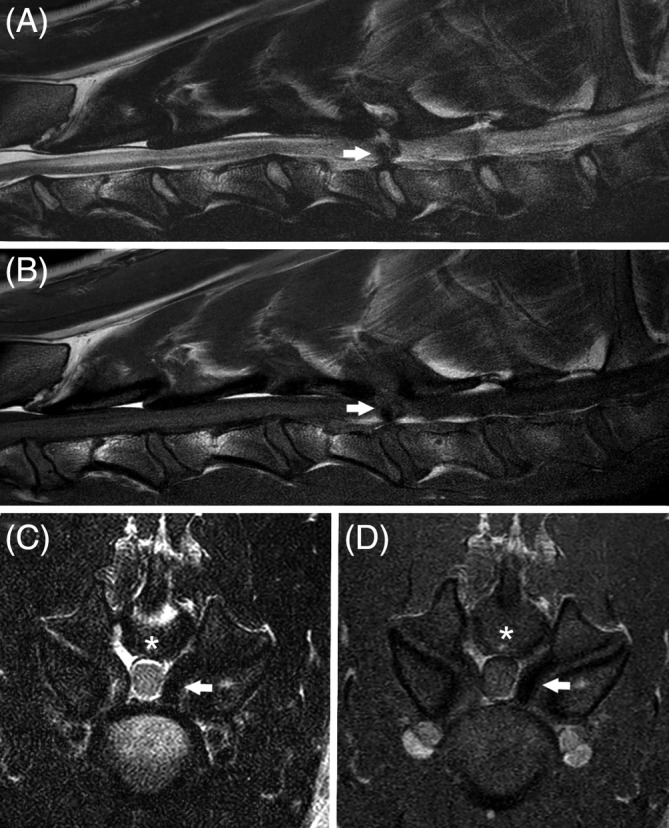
Parasagittal T2‐weighted (A) and T1‐weighted (B), transverse T2‐weighted (C) and T1‐weighted (D) magnetic resonance imaging of a 12‐month‐old Great Dane with cervical spondylomyelopathy because of osseous proliferation of the articular processes, more prominent on the left (arrows). Also, note the hypertrophy of the ligamentum flavum (asterisk)
